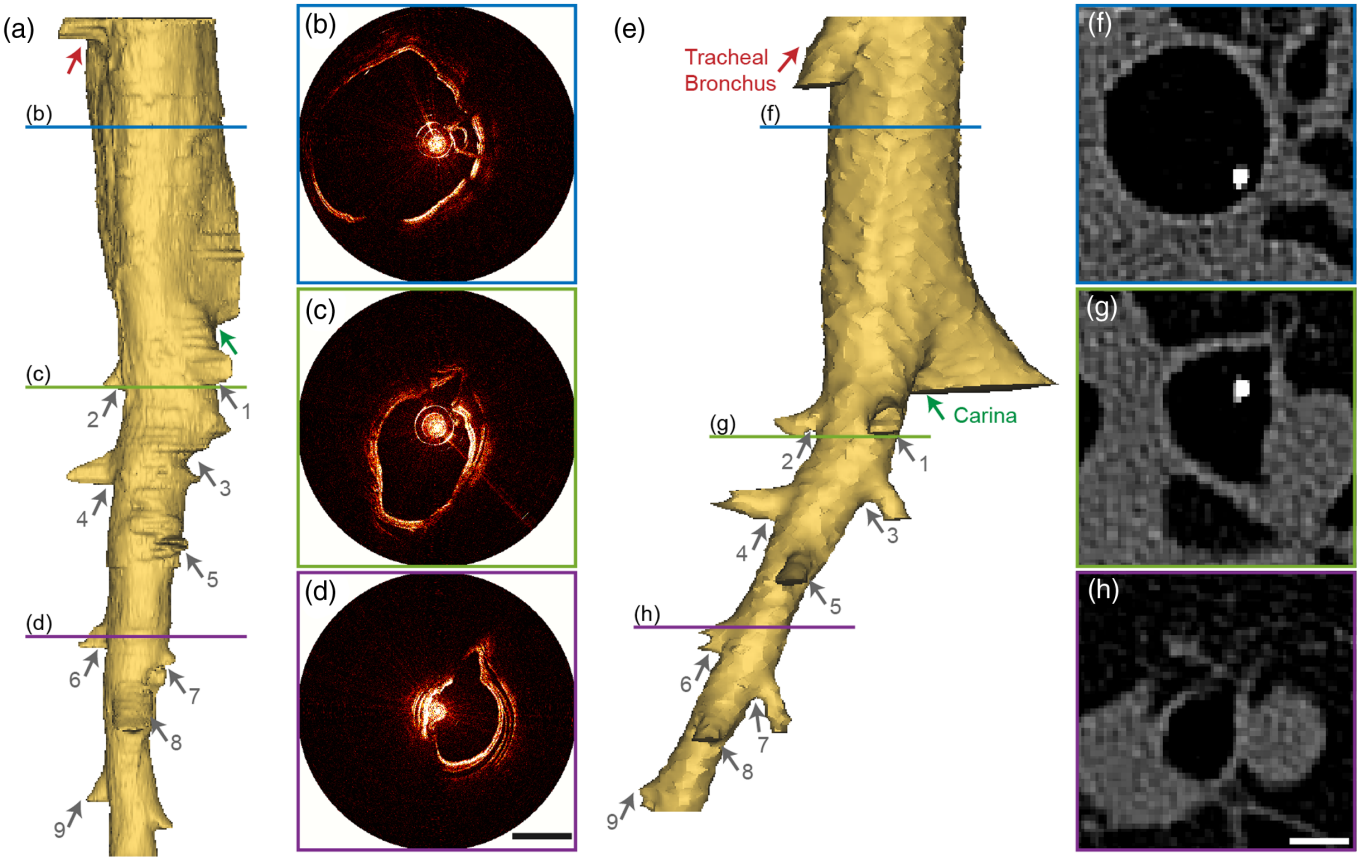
Volumetric data from aOCT scans compared with CT results; (a) 3-D reconstruction from a ∼10-cm-long pullback aOCT scan; (b)–(d) aOCT polar images at the sites indicated by the colored bars on (a); (e) CT derived 3-D volume; (f)–(h) axial CT images corresponding to (b)–(d); labeled arrows on the 3-D volumes indicate corresponding features; scale bars=5  mm.
